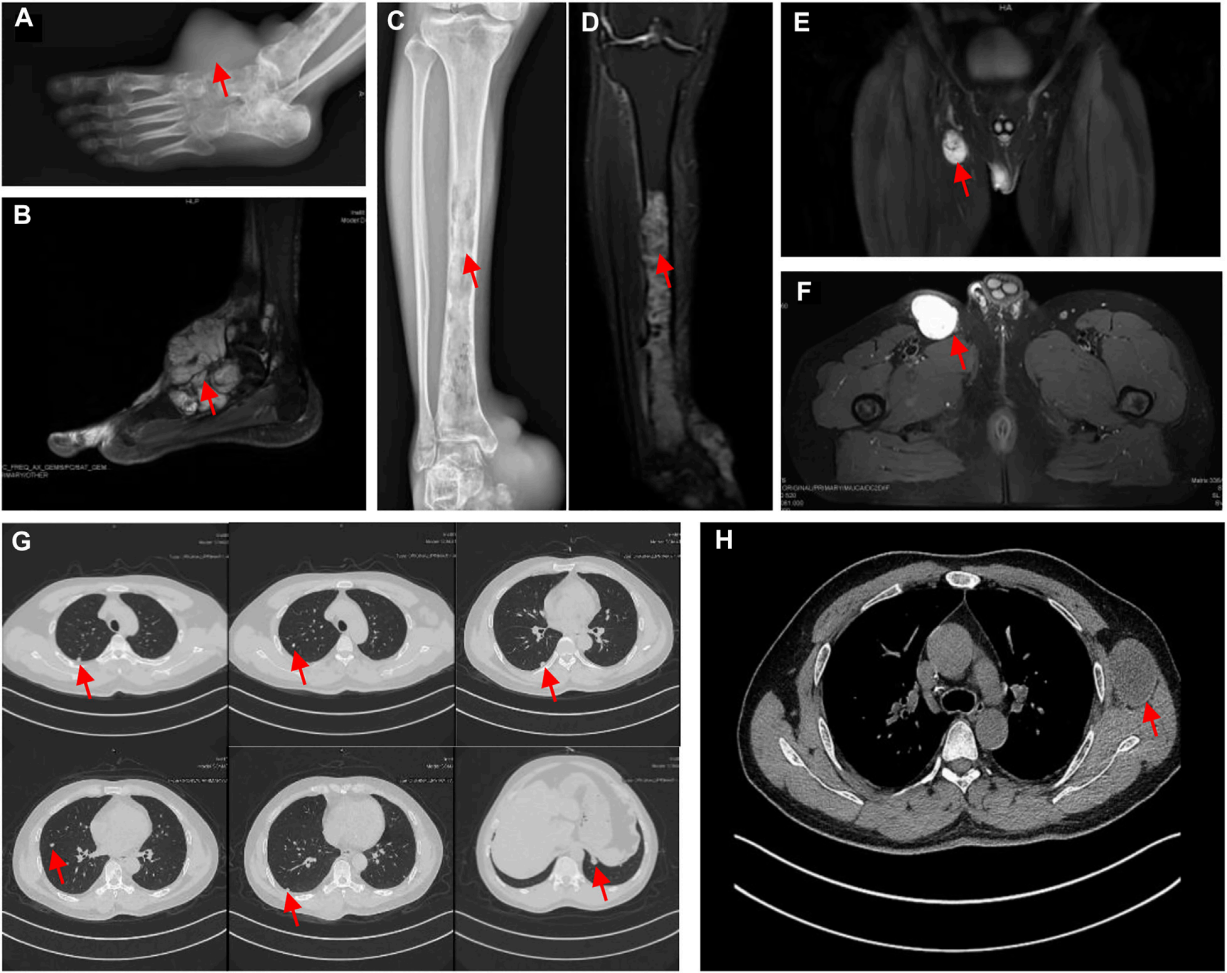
(A) The radiograph showed an increase in size compared with Figure 1A and implication of the tarsus and talus; the radiograph revealed that pathological fracture in the middle of the right femur with a heterogeneous low signal (red arrow) in the medullary cavity. (B) T2-weighted MRI showed a heterogeneous high homogeneous signal in T2WI with a clear border. (C) and (D) the X-ray and MRI revealed the metastasis in the tibia and fibula. Consecutive bone destruction was seen in the middle and lower segment of the tibia and the distal fibula. (E) and (F) The MRI (coronal and transverse sections) displayed the metastasis in right inguinal lymph nodes (G) CT scan of the chest showed the pulmonary metastasis. (H) CT showed the left axillary lymph node metastasis.
